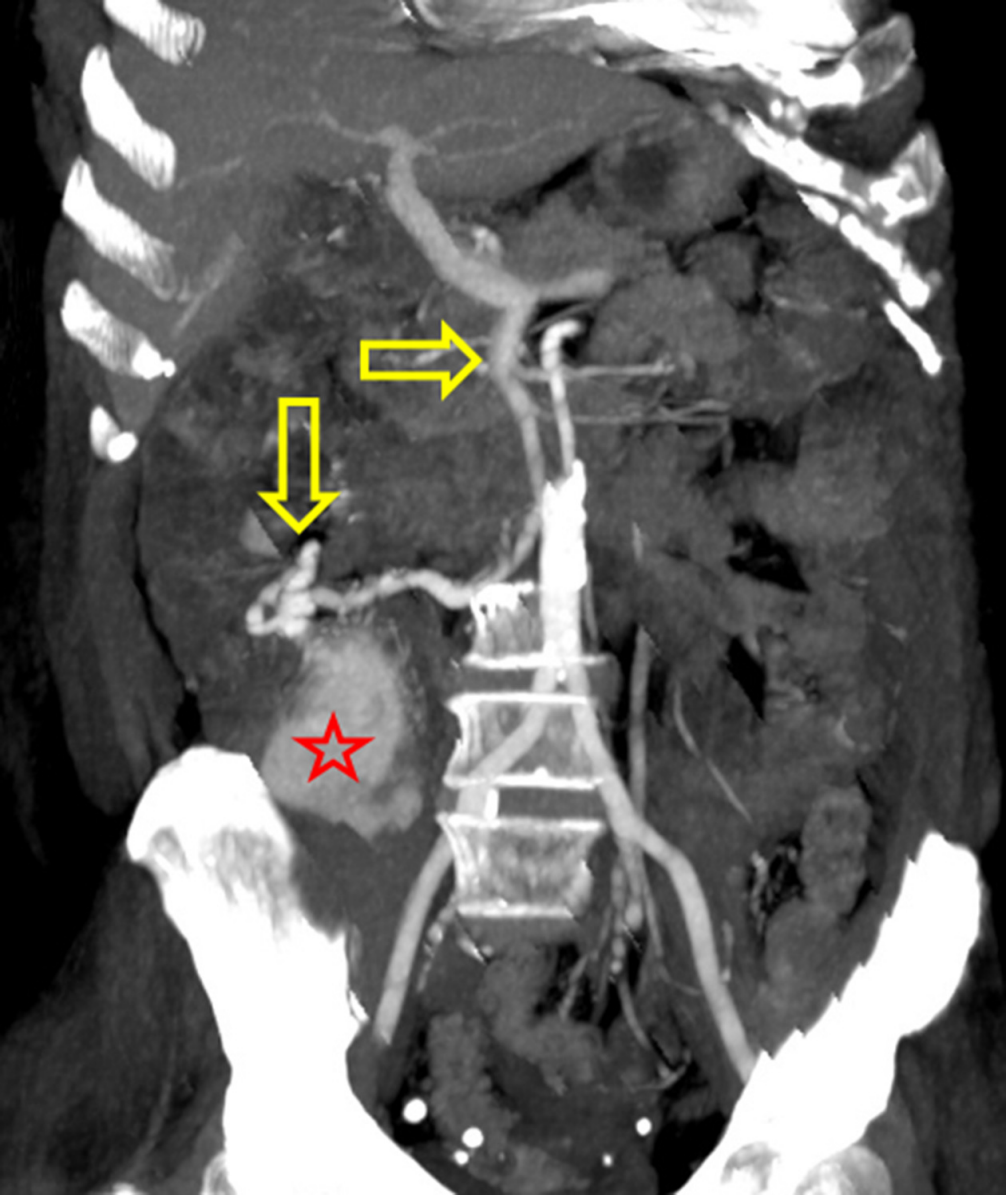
Maximum intensity projection of coronal contrast-enhanced CT scan in arterial phase. Exophytic ileal mass (red star), drained by aberrant hypertrophic vascular structure originating from the portal vein (yellow arrows).
